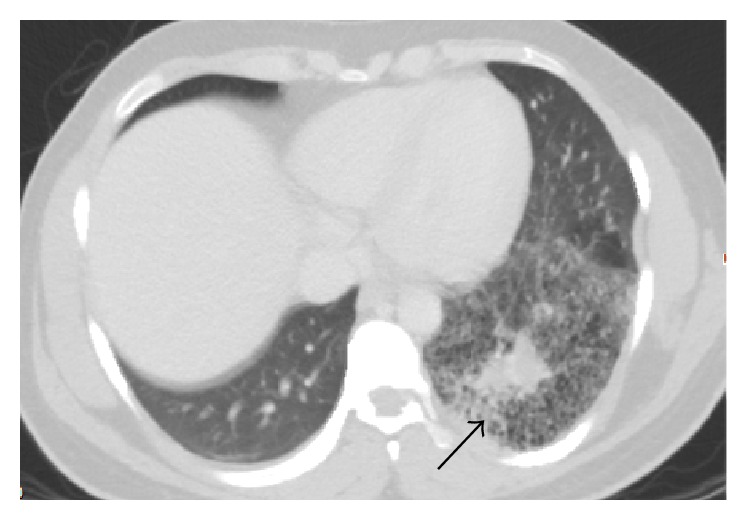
CT scan 4 months ago showing infiltrates in the left lower lung.
